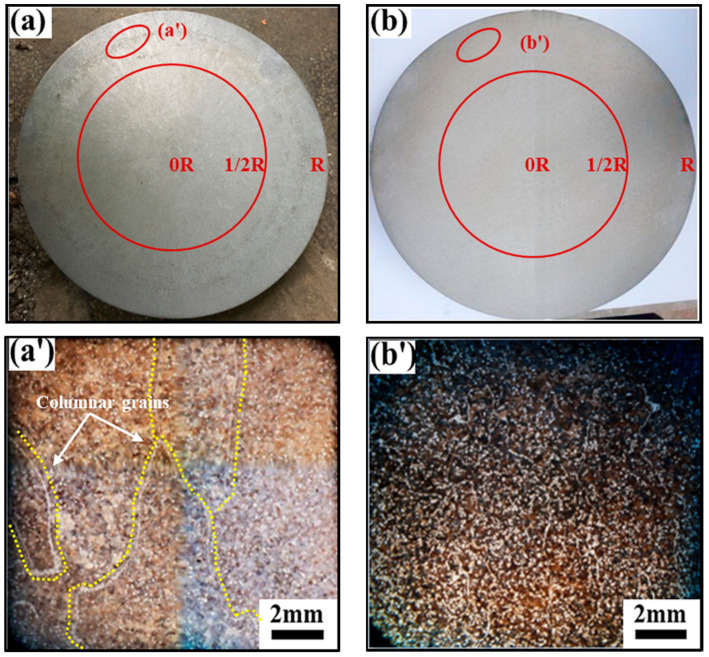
Macro- and microstructure of the ingot: (a,a′) without ultrasonic; (b,b′) with ultrasonic.
